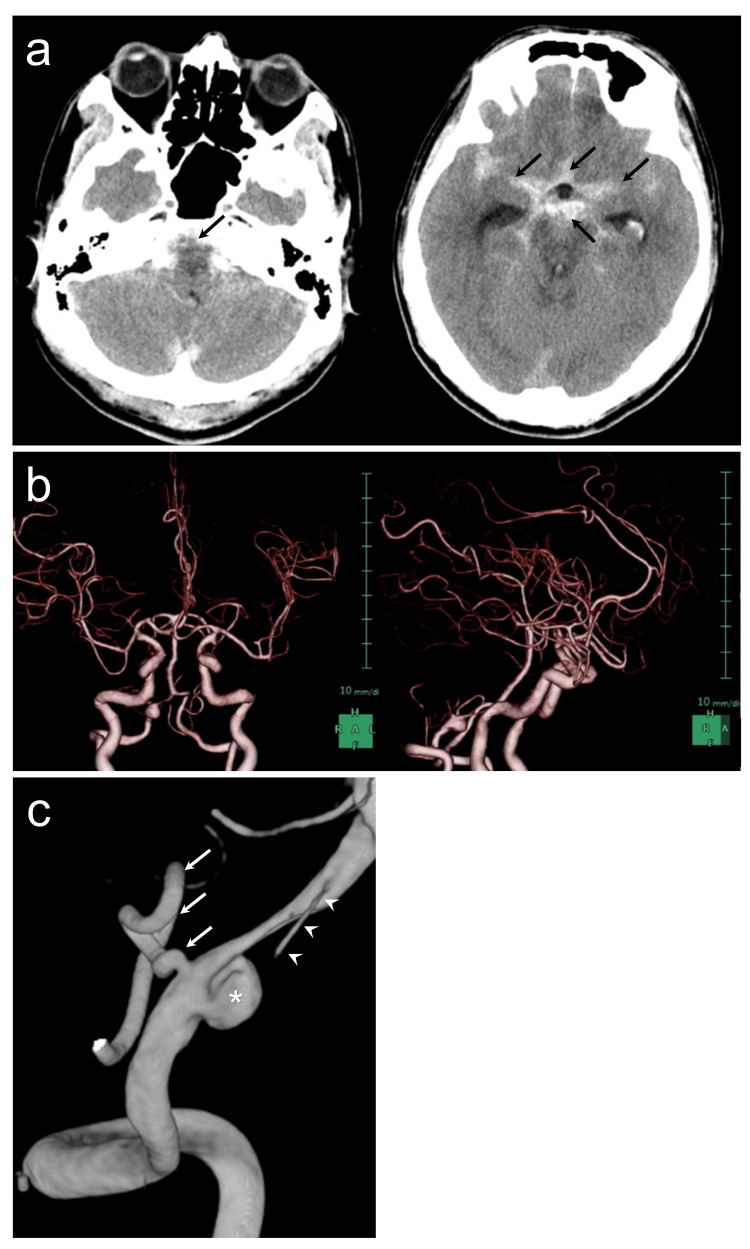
Preprocedural imaging of the case. (a) Preprocedural CT shows subarachnoid hemorrhage in the basal cistern, prepontine cistern, and sylvian fissure (black arrows). (b) Preprocedural CT angiography shows the right vertebral artery dissecting aneurysm (VADA) involving the posterior inferior cerebellar artery (PICA). The left vertebral artery (VA) developed almost equally compared to the right VA. (c) Preprocedural three-dimensional rotational angiography reveals right VADA (white asterisk). The PICA (white arrows) arises from dorsal of the aneurysm, and the anterior spinal artery (ASA, white arrowheads) arises distal to the aneurysm. Stenosis between the aneurysm and the ASA origin is also suspected of dissection.
